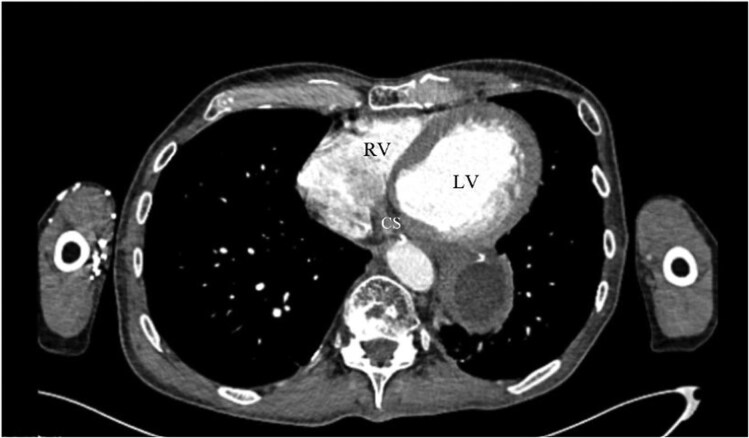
Preoperative CT showing distorted thoracic anatomy, precluding epicardial access.
